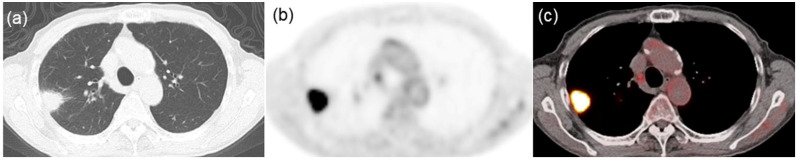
Representative images from an 85-year-old man with lung squamous cell carcinoma characterized by high PD-L1 expression. (a) CT image displaying a lung mass in the right upper lobe. (b) FDG PET and (c) PET/CT fusion images exhibiting intense tracer uptake within the tumor (SUVmax = 21.42, LGRE = 0.0006, SRLGE = 0.0006, LRHGE = 2083, LGZE = 0.0007, HGZE = 1919, and SZLGE = 0.0006).
